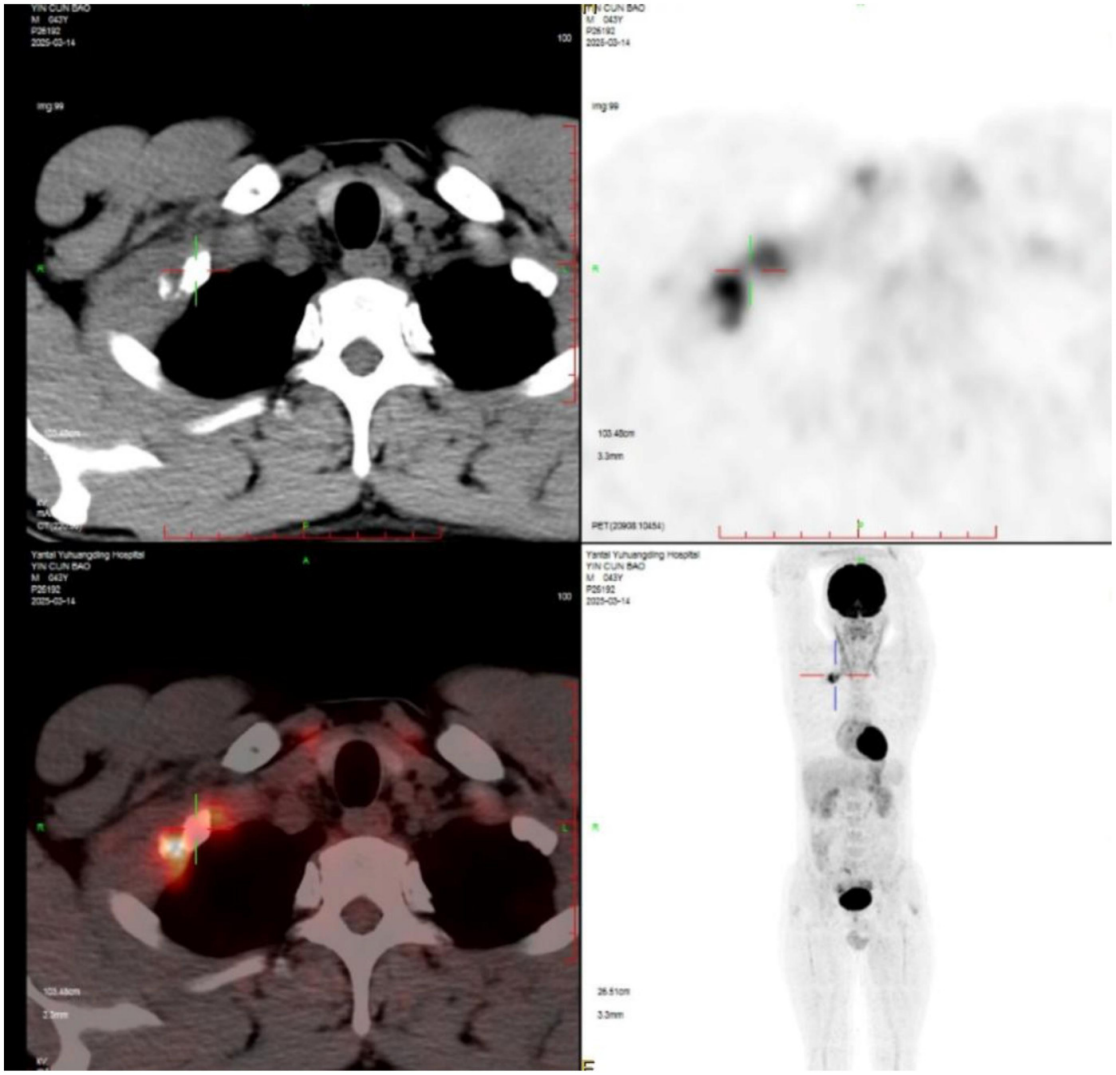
PET-CT demonstrates a single lesion in the middle part of the first right rib with an SUVmax of 11.1.
